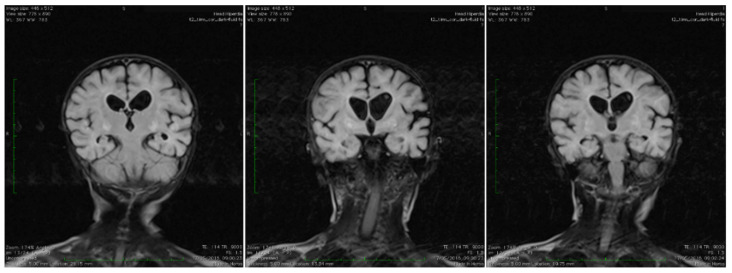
Coronal T2 TIRM dark fluid MRI sequences revealing bilateral basal ganglia hyperintensities and cerebral atrophy.
